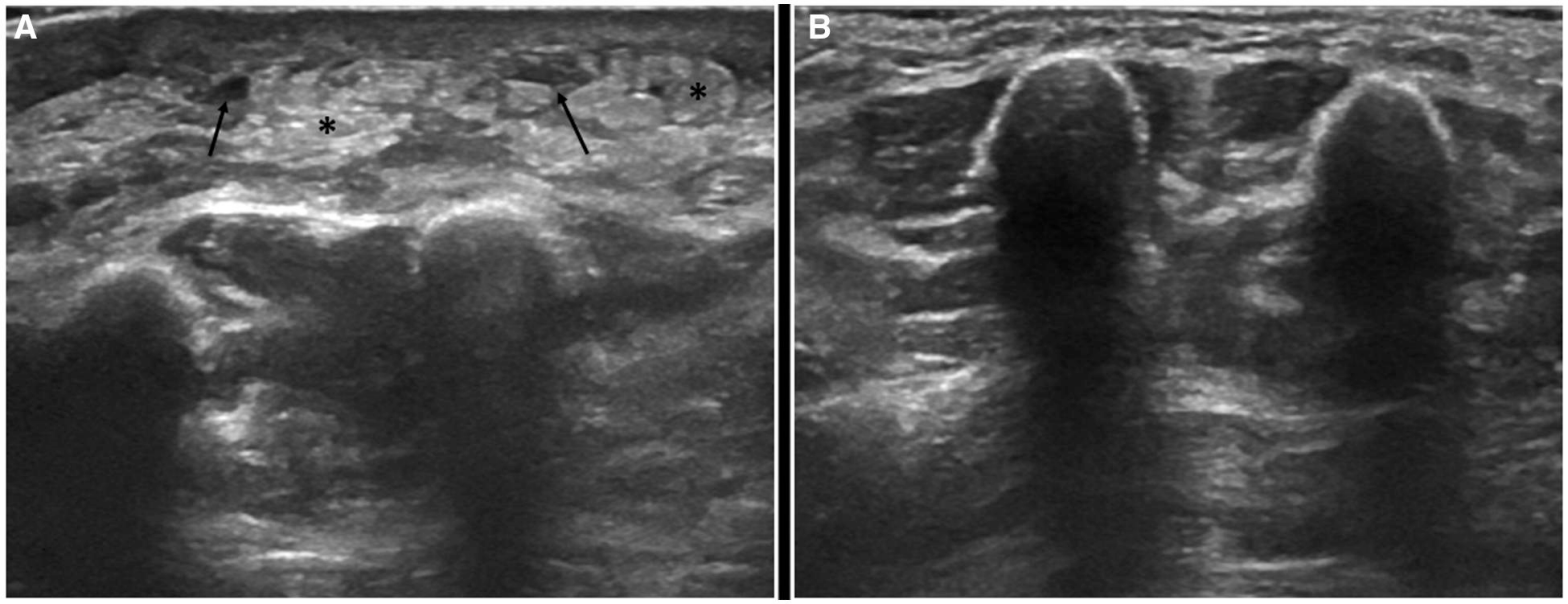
Ultrasound of the right limb (A) at the mid-forearm level in a patient operated for right breast cancer, post radiation therapy, 6 months back, with increased hyperechogenicity and hypertrophy of the subcutaneous fat (asterisk), with associated interspersed fluid clefts in between the fat lobules (arrows). Findings are consistent with grade 1 SEG. Note the normal thickness of the dermis as well as the subcutaneous tissue in the contralateral limb (B).
